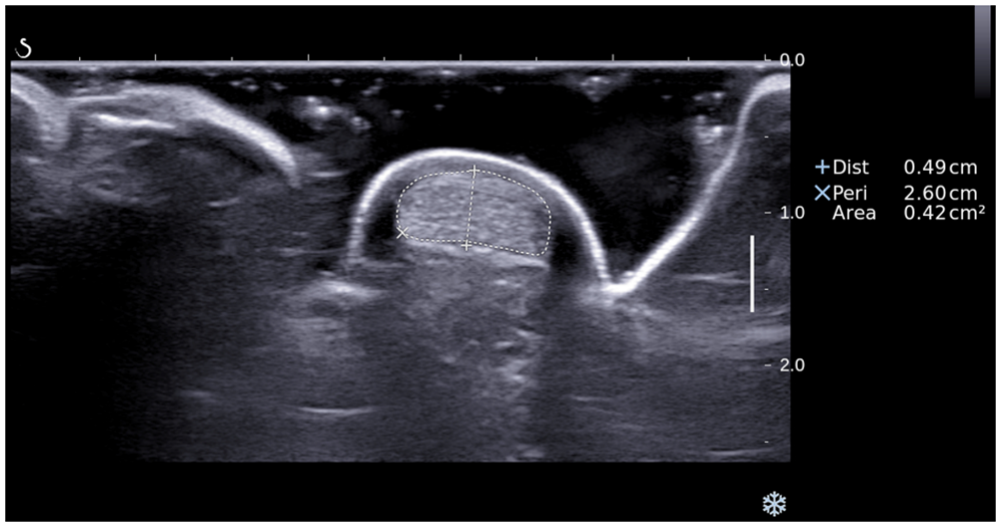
A transverse grey scale sonogram of Achilles tendon shows the measurement of the thickness (caliber +) and cross-sectional area (caliber ×) of the tendon.
